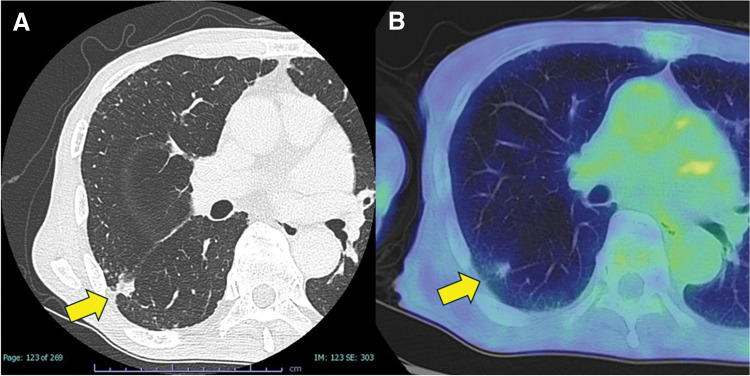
(A) A chest CT scan revealed a 2.2 × 1.4-cm nodule in the right lower lobe (S6) with an unclear border and a tendency to grow, plus suspected infiltration into the right upper lobe (S2). Features consistent with malignancy, such as pleural indentation and focal pleural thickening, were observed. (B) PET showed a minimal accumulation of FDG in the nodule, with the SUVmax of 1.0 in the early phase.
